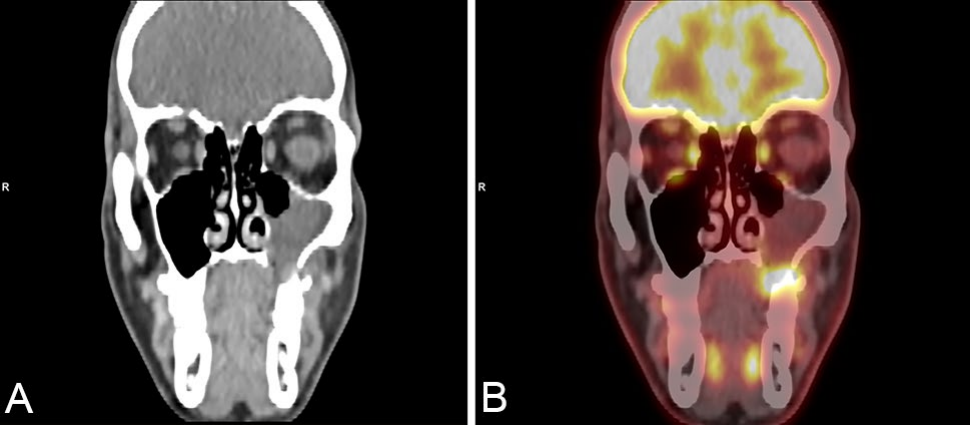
A Coronal CT scan of the paranasal sinuses showing a large bone defect and opacification of the left maxillary sinus. B FDG-PET/CT showing an intensely metabolically active tumor with osseous destruction of the left maxillary sinus
